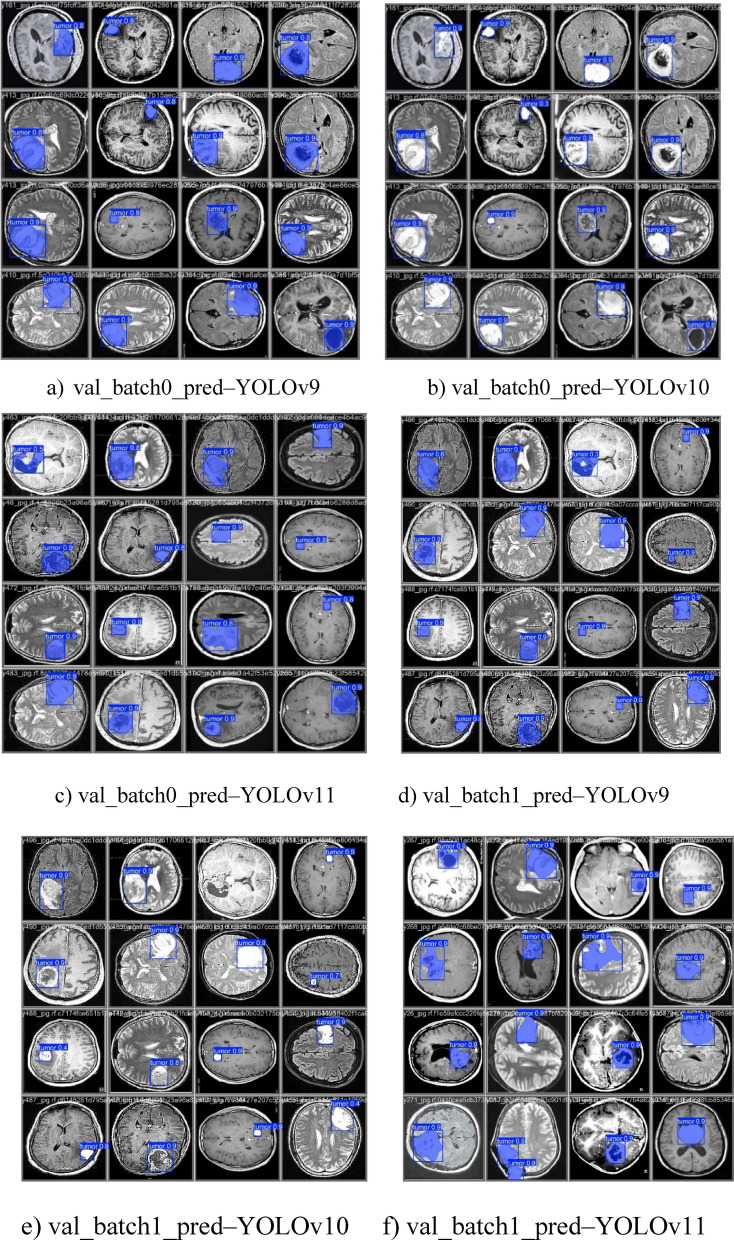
Visualization of tumor detection on MRI images using YOLO models.
